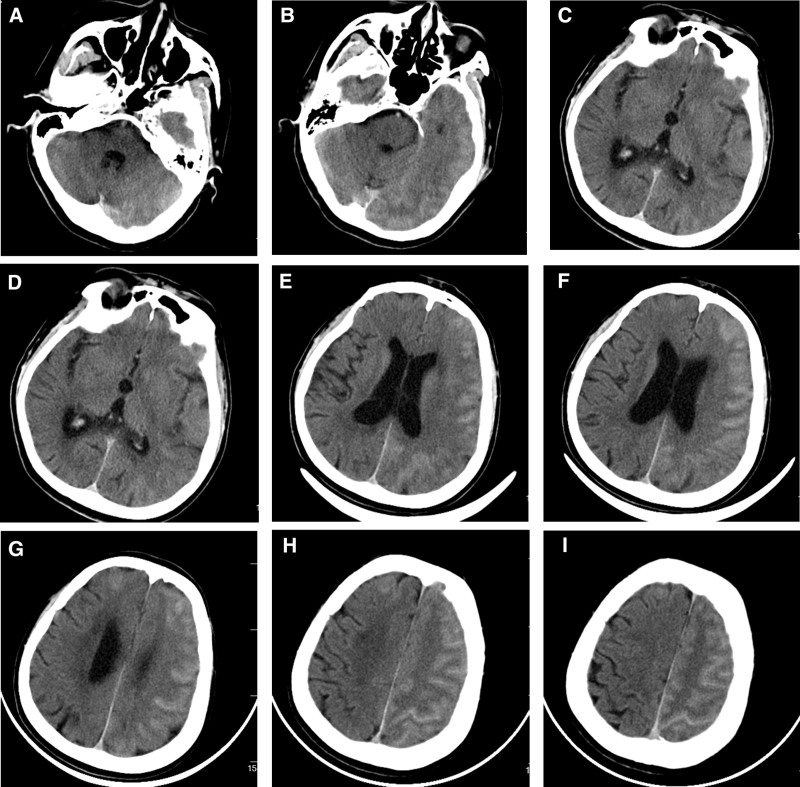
CT scan of the brain immediately after stenting when the patient developed symptoms. (A–I) Multiple high-density strips were observed in the left cerebral hemisphere, part of the right frontal sulcus, cerebral falx, and cerebellar curtain. Edema of brain tissue was also observed. CT = computed tomography.
